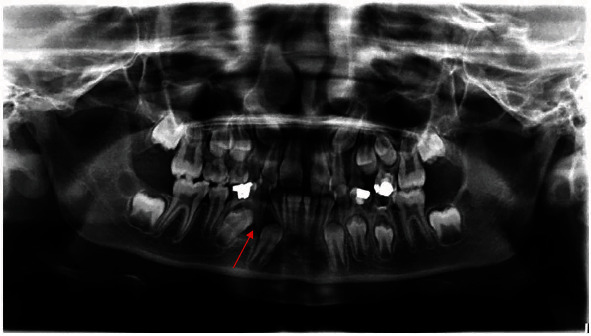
Preoperative panoramic radiograph (red arrow).
